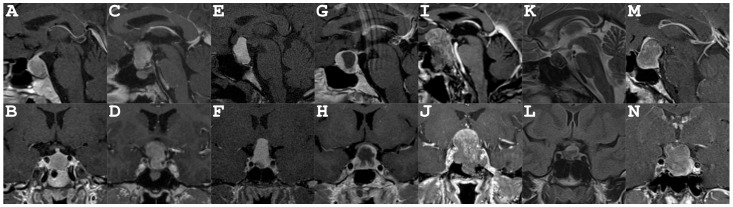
Initial MRI of Patients 2–7 (cf Table 1): (A,B): Patient 2, (C,D): Patient 3, (E,F): Patient 4, (G,H): Patient 5, (I,J): Patient 6, (K,L): Patient 7, (M,N): Patient 8. Overall lesions showed both intralesions and interlesional heterogeneity.
